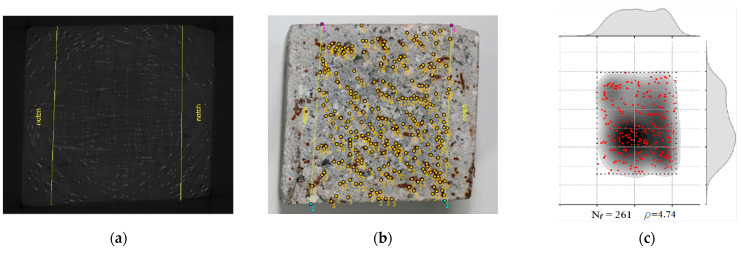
Identification of fibers of a shear cross-section: (a) CT image; (b) fiber position and counting: labels 1 and 2 are the top and bottom points, respectively, defining the limits of the shear section, and label 3 corresponds to every fiber position (c) distribution and density of fibers.
